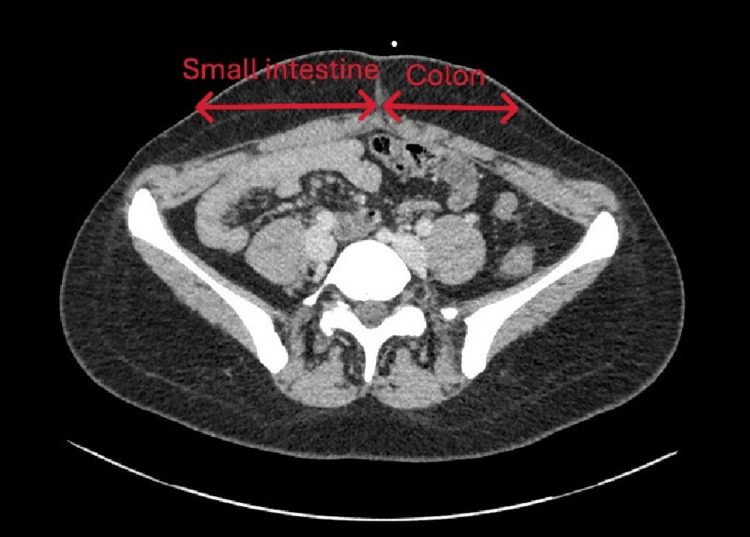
Abdominal CT scan showing the small intestine located on the right side of the abdomen
